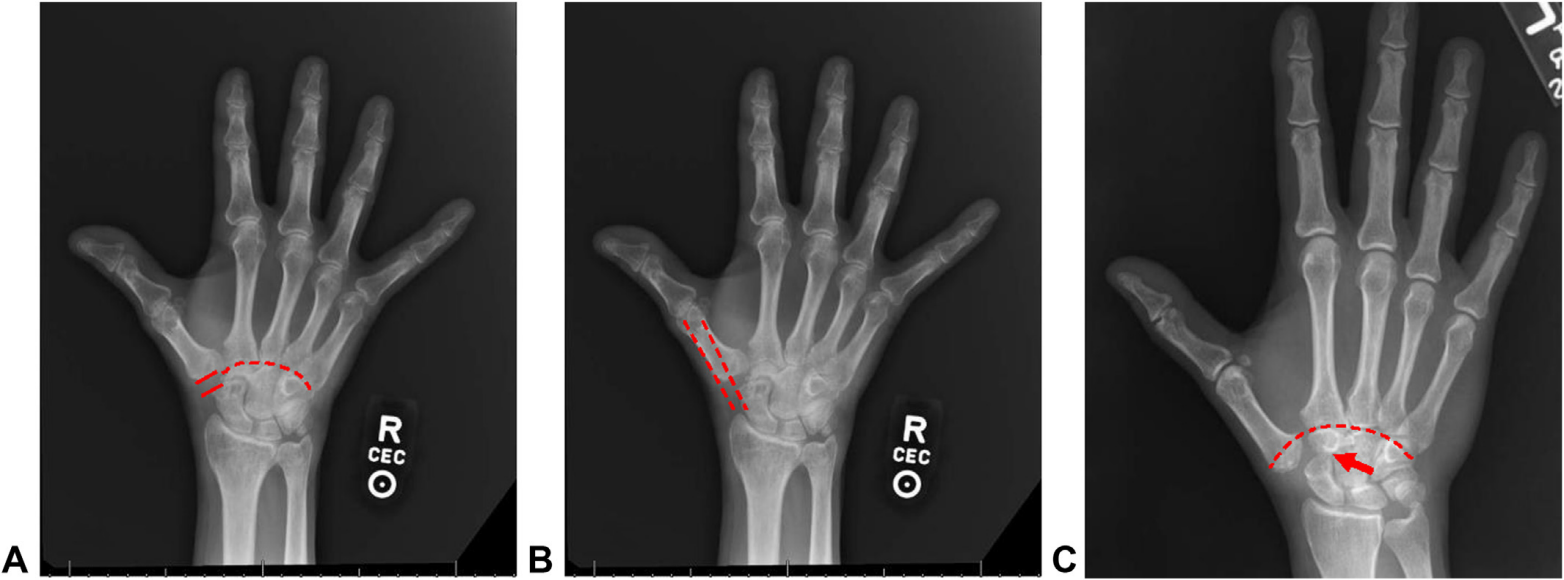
Measurement of radiographic features in thumbs undergoing revision arthroplasty. Metacarpal instability is reflected in trapezial space height (A), percent radial subluxation of the first metacarpal base (B), and migration of the thumb metacarpal base (dashed lines in A, C). Trapezial space height is measured from the base of the thumb metacarpal to the distal tip of the scaphoid. Radial subluxation of the thumb metacarpal is measured by extending a line along the radial cortex of the thumb metacarpal shaft, translating it ulnarly until meeting the border of the scaphoid, and dividing this distance by the width of the thumb metacarpal base. Migration of the thumb metacarpal base is measured by Shenton’s line, which is depicted as a continuous (dashed line, A) or discontinuous (dashed line, C) line of curvature connecting the proximal limit of the index and thumb metacarpals. Scaphotrapezoid arthritis (C, arrow) is evidenced by subchondral sclerosis and osteophytes. In the two leftmost radiographs, R refers to right-sided and CEC refers to using radiographic quality criteria developed by the Commission of European Communities (CEC).
